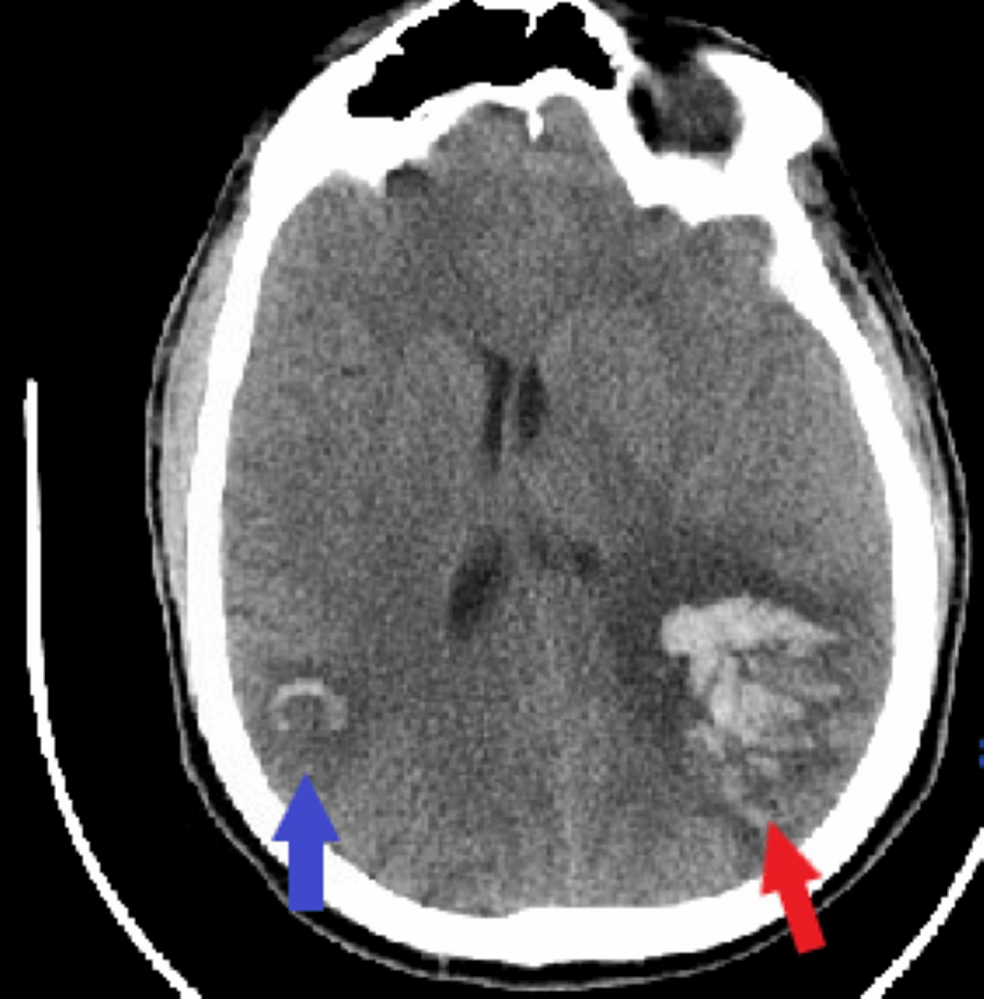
Computed tomography of the head (axial view). The blue arrow shows the right parietal lobe intraparenchymal hematoma, and the red arrow shows the left frontoparietal intraparenchymal hematoma.
